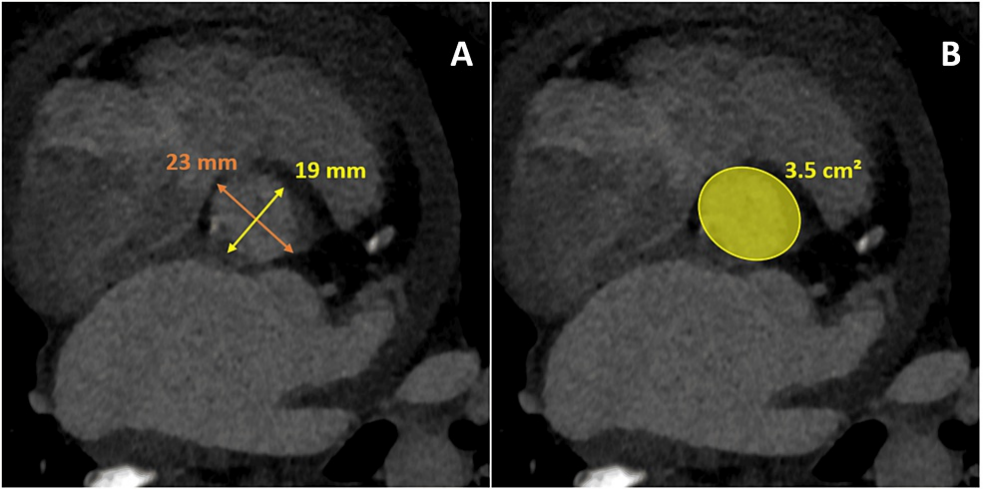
An example of aortic valve annulus measurement on CT in a 63-year-old female patient with severe aortic stenosis. Image A shows that the maximum transverse diameter was 23 mm and the minimum transverse diameter was 19 mm, resulting in a mean transverse diameter of 21 mm. Image B shows that the annulus area of the aortic valve was 3.5 mm, yielding an area-derived diameter of 21.1 mm.
